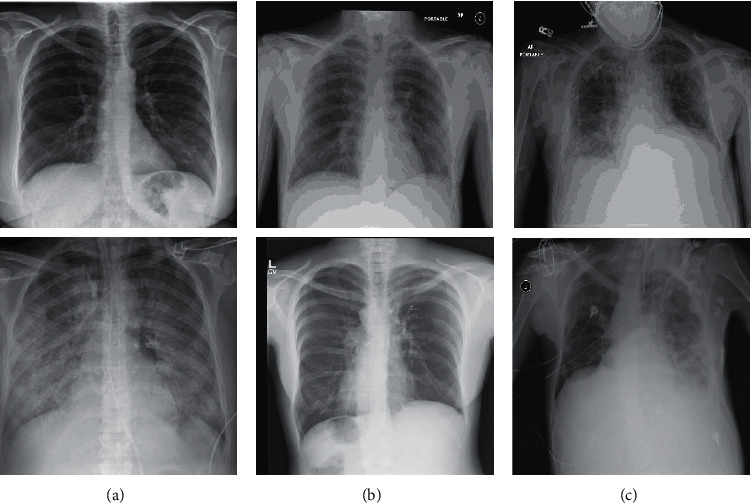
Representation of chests X-ray in COVID-19 patients (a), No-findings (b), and patients with Pneumonia (c).
